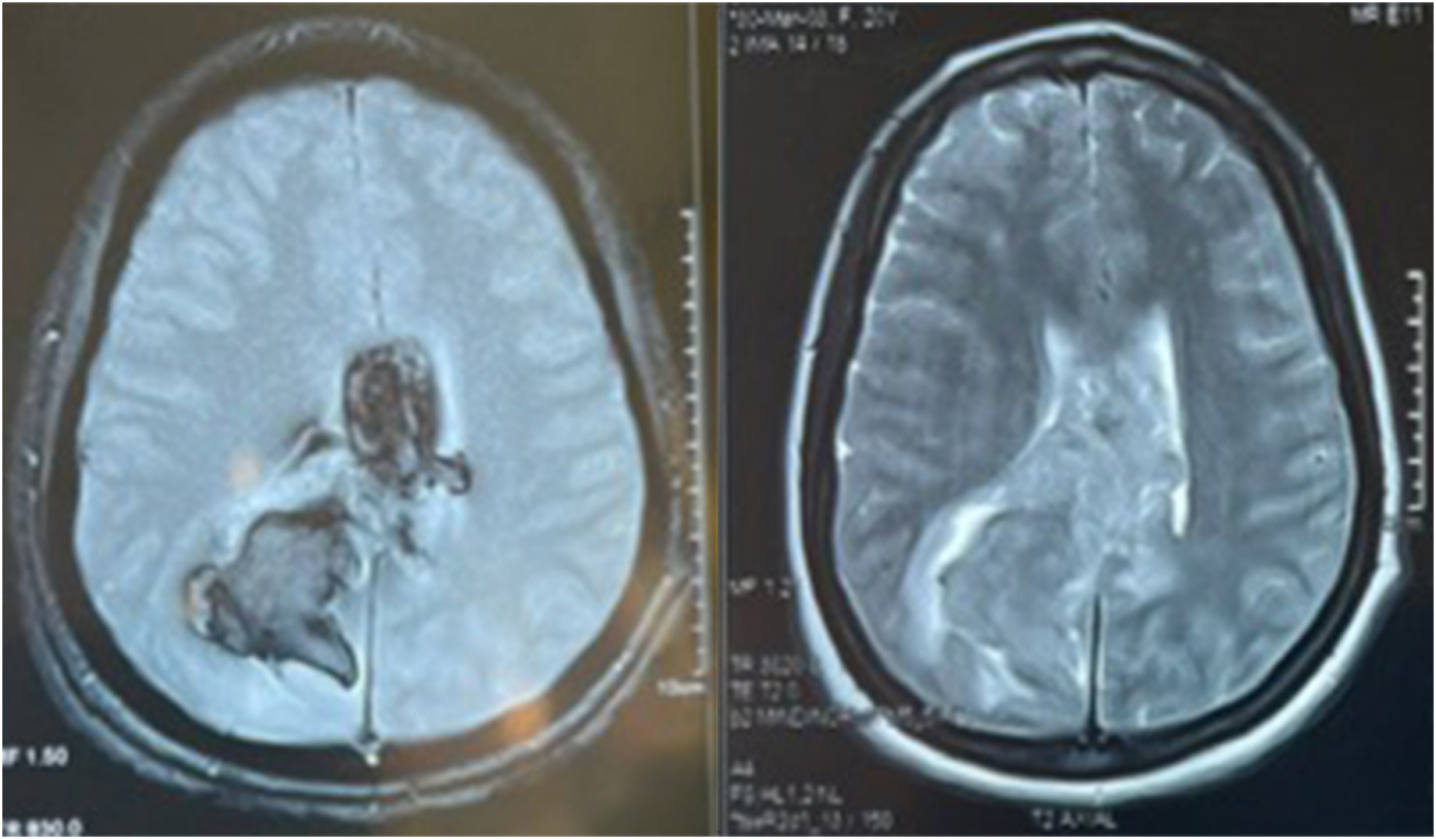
Case 3. MRI of the brain showed a large subacute right temporo‐occipital subependymal hemorrhage, which measures approximately 5.5 × 4.2 cm with intraventricular extension and exhibiting minimal perilesional edema.
